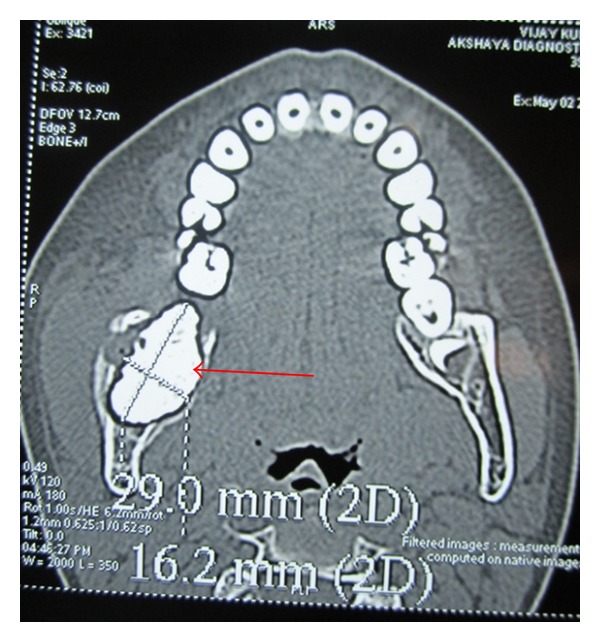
Axial CT scan section showing the extent of the lesion anteroposteriorly.
